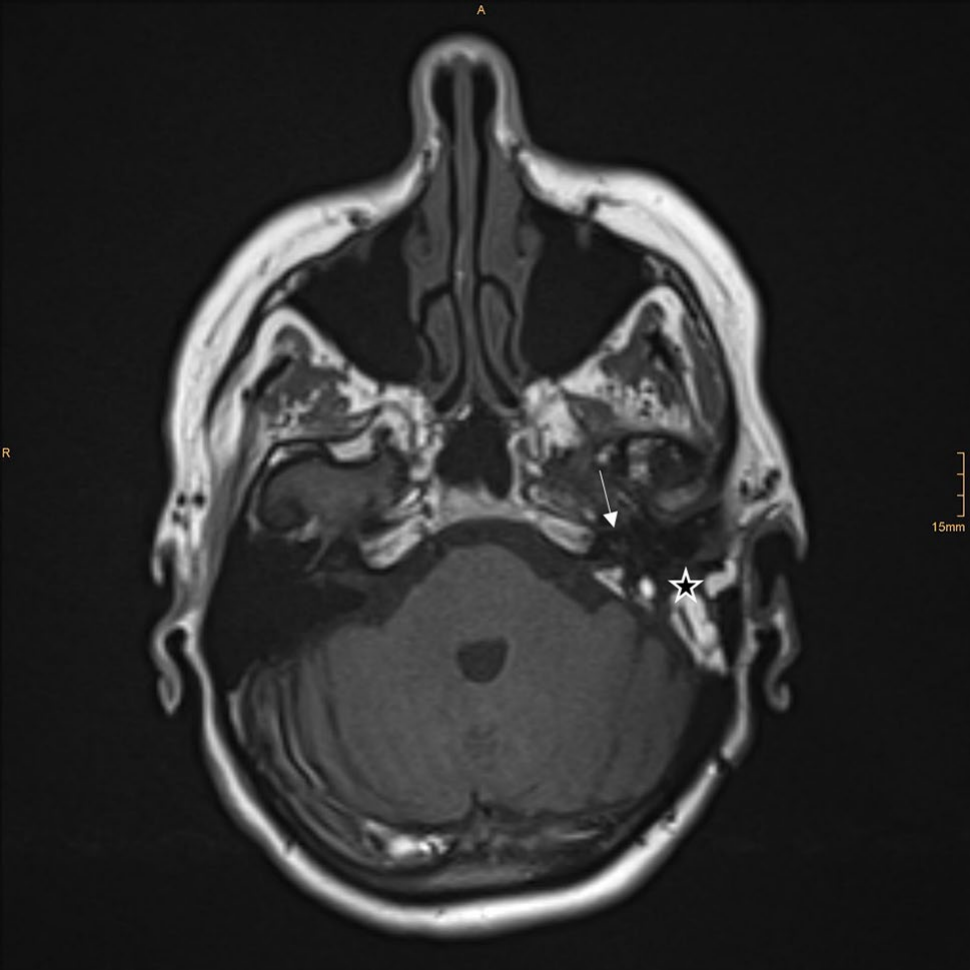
MRI T1, axial, postoperative image. The arrow points at the cochlea, the star is placed in the previous mastoid region showing postoperative changes. This image was rated as Grade 3—excellent image quality
